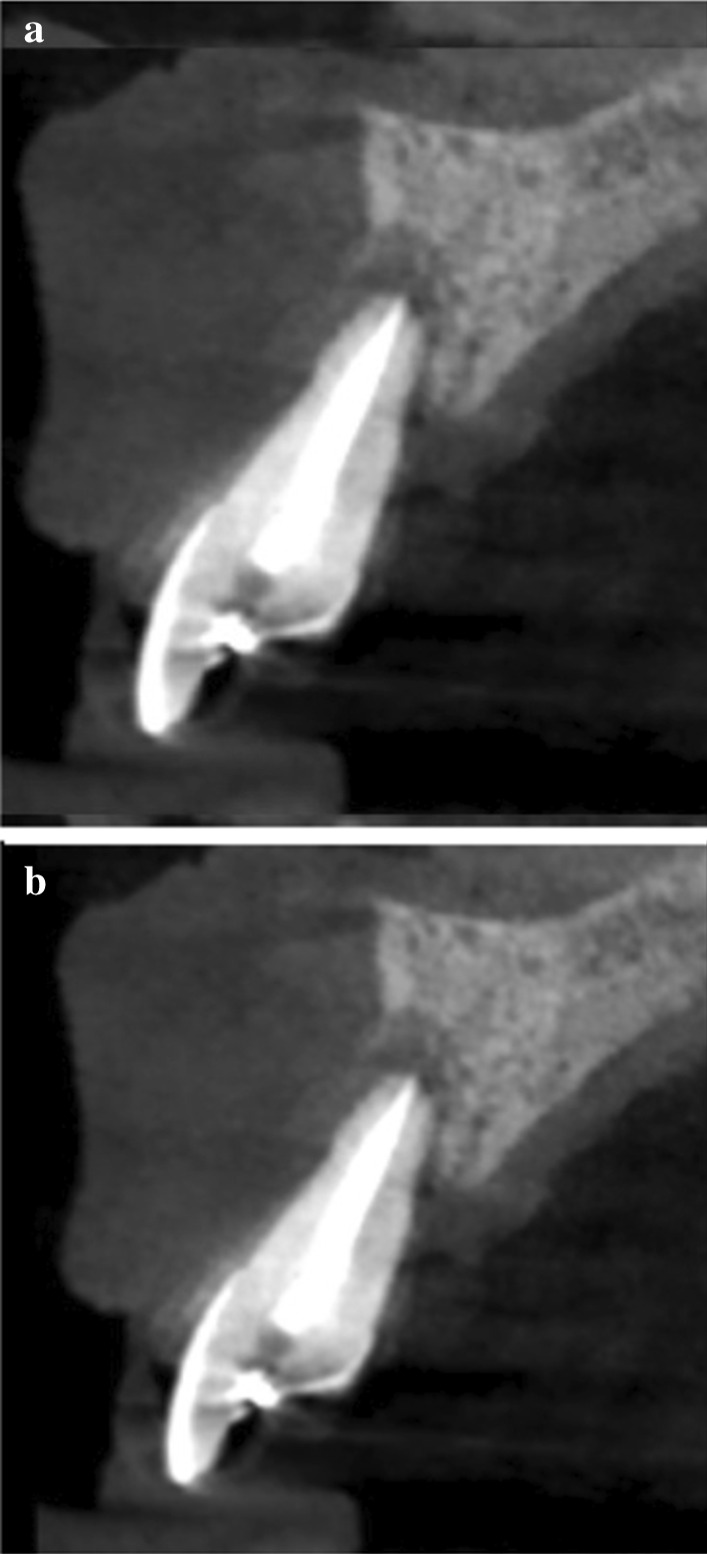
Baseline CBCT scan of upper middle incisors, (a) upper right first incisor, (b) upper left first incisor
